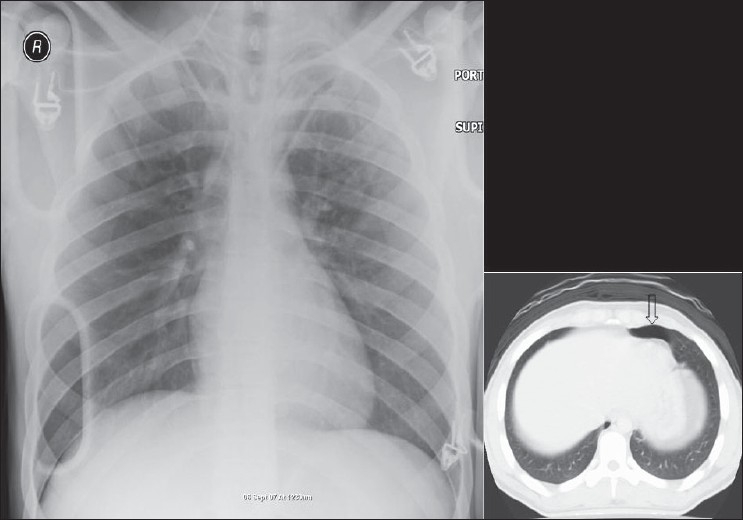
AP chest radiograph may miss a small pneumothorax. One such example is seen above; note the small collection of air anteriorly on the axial CT, representing a small pneumothorax
